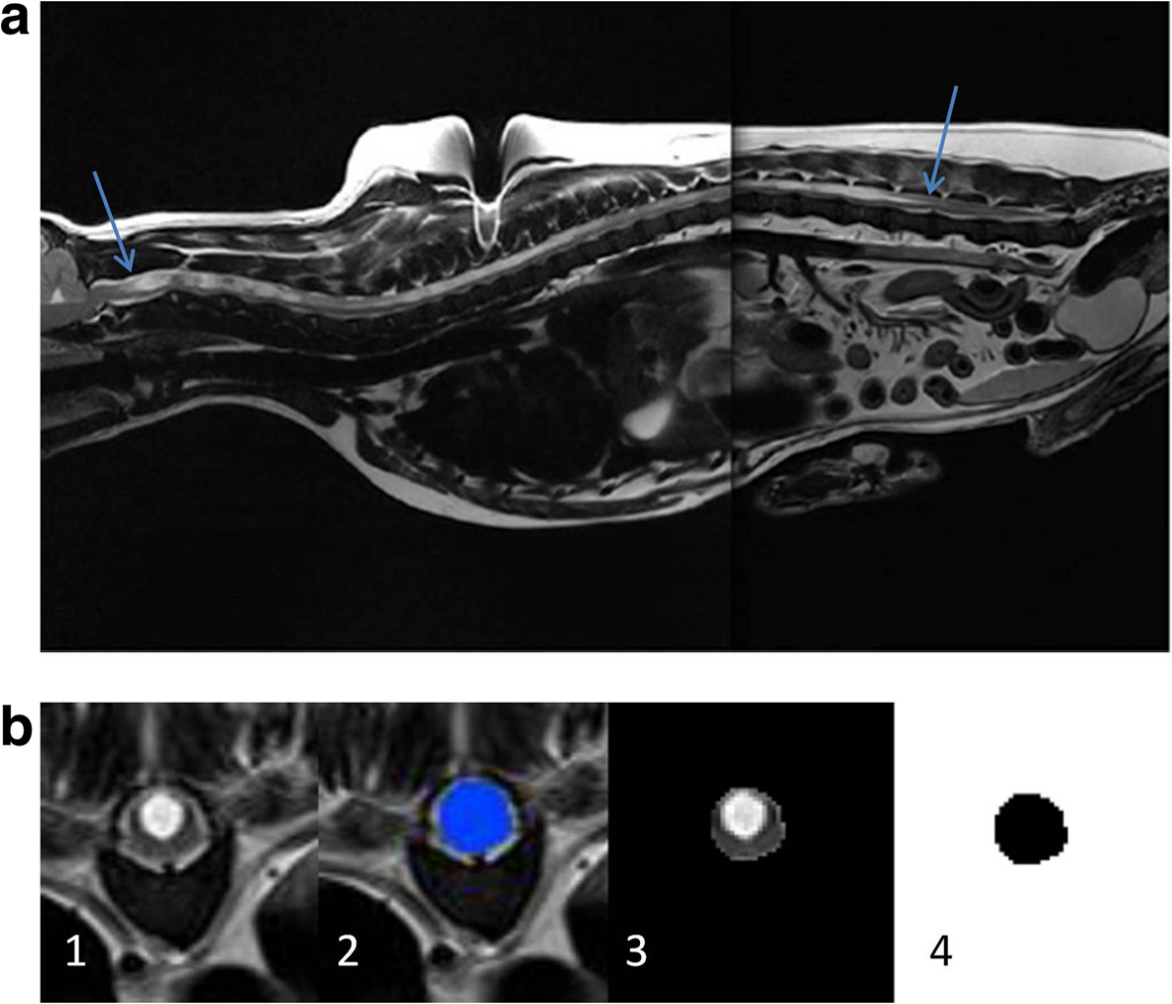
a T2-weighted mid-sagittal MRI of a CKCS suffering from a large syrinx (syrinx ends indicated with arrows). b The geometry reconstruction process. 68 transverse plane scans were obtained (1). Each anatomical layer was segmented to create a mask (2). The mask was filtered out (3) and then binarised (4) to create a pure black and white image
